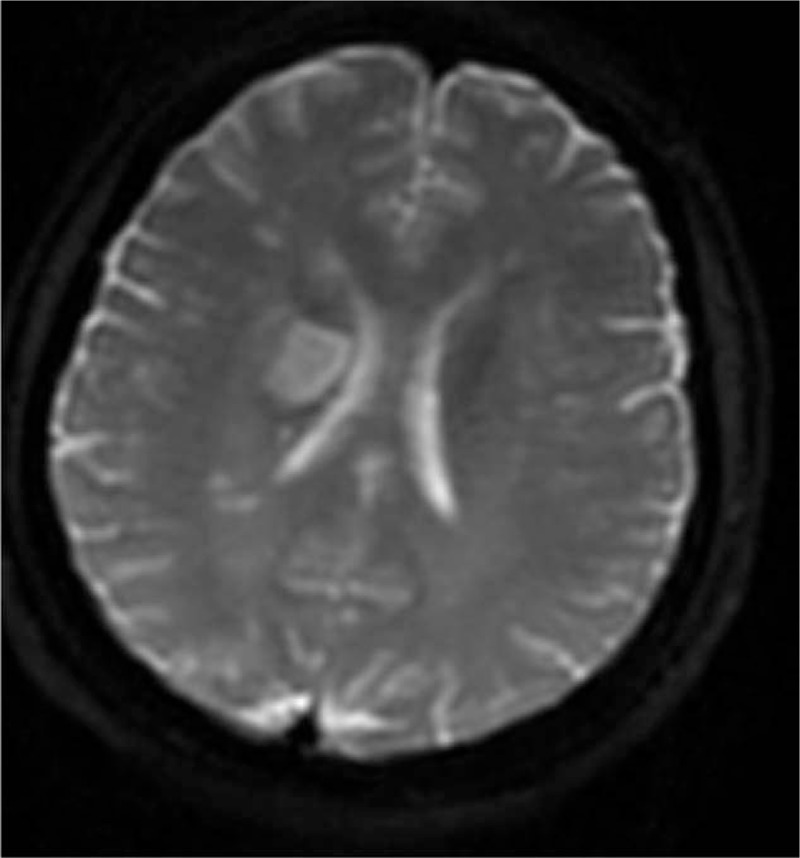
Magnetic resonance image of the patient.
